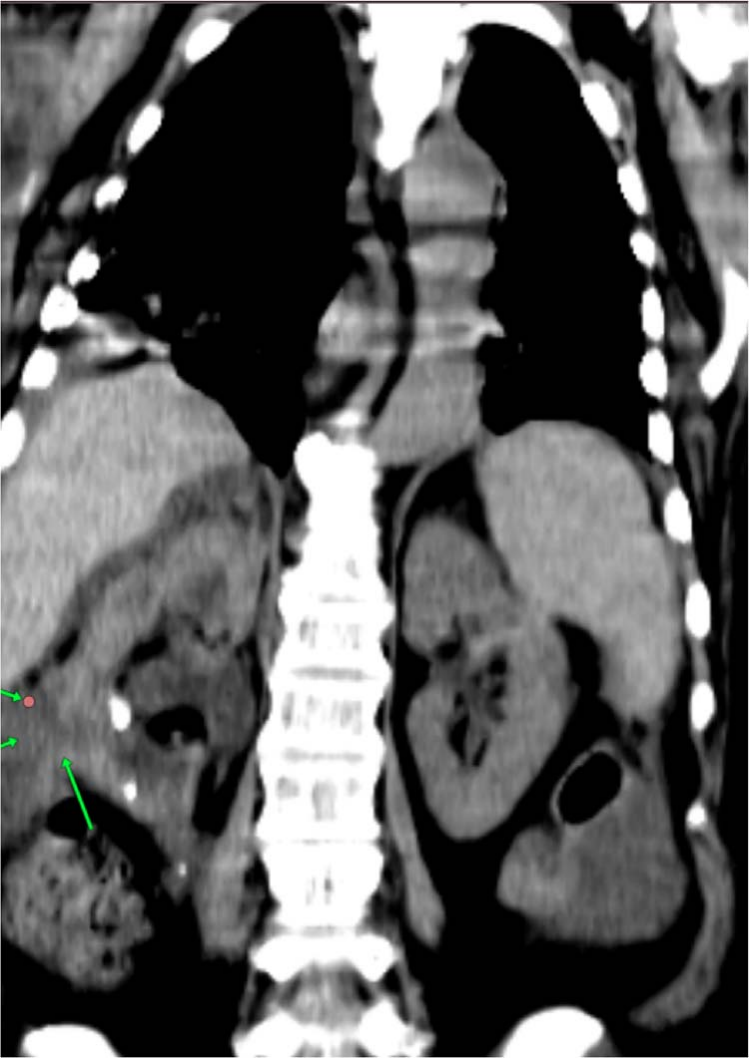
None enhanced computed tomography show kidney stones, ascites and swollen right kidney with the perinephric collection.
